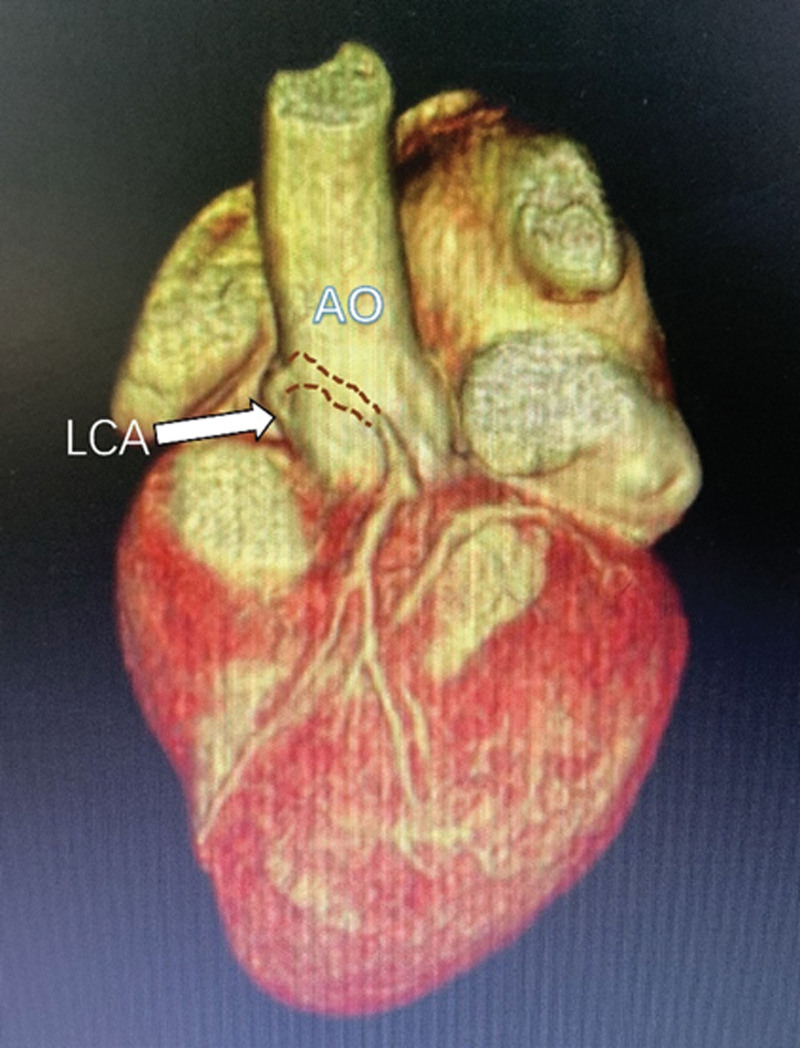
Coronary CTA: LCA is originated from the opposite sinus, and exhibited intramural course.
